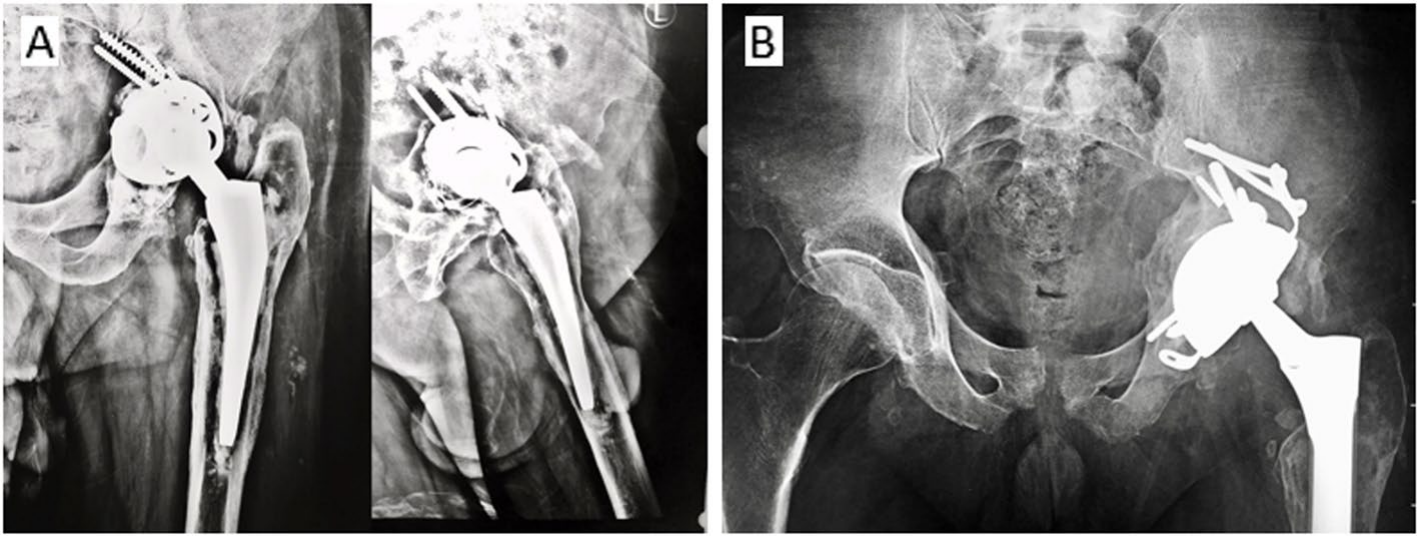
A Preoperative x-ray for a loose cemented cup and Muller ring from an earlier revision THA Paprosky IIIB bone defect (B) 4 years postoperative x-ray of a stable Coptos cup and incorporated bone graft that was impacted in the medial defect
